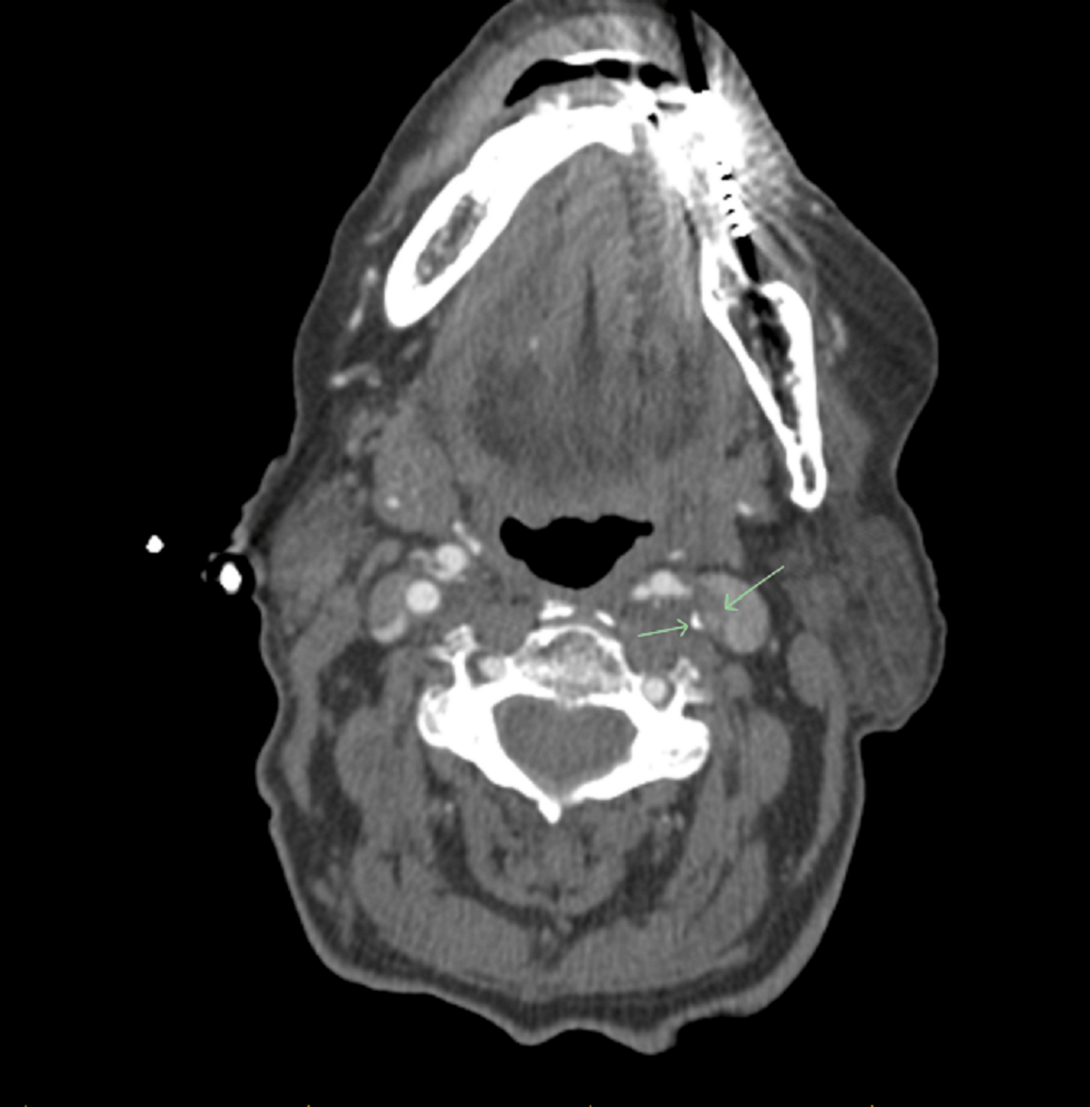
Coronal section of the CT angiogram with green arrows pointing to the left carotid artery stenosis Green arrows demonstrating 70% stenosis of the left internal carotid artery. CT: computed tomography
